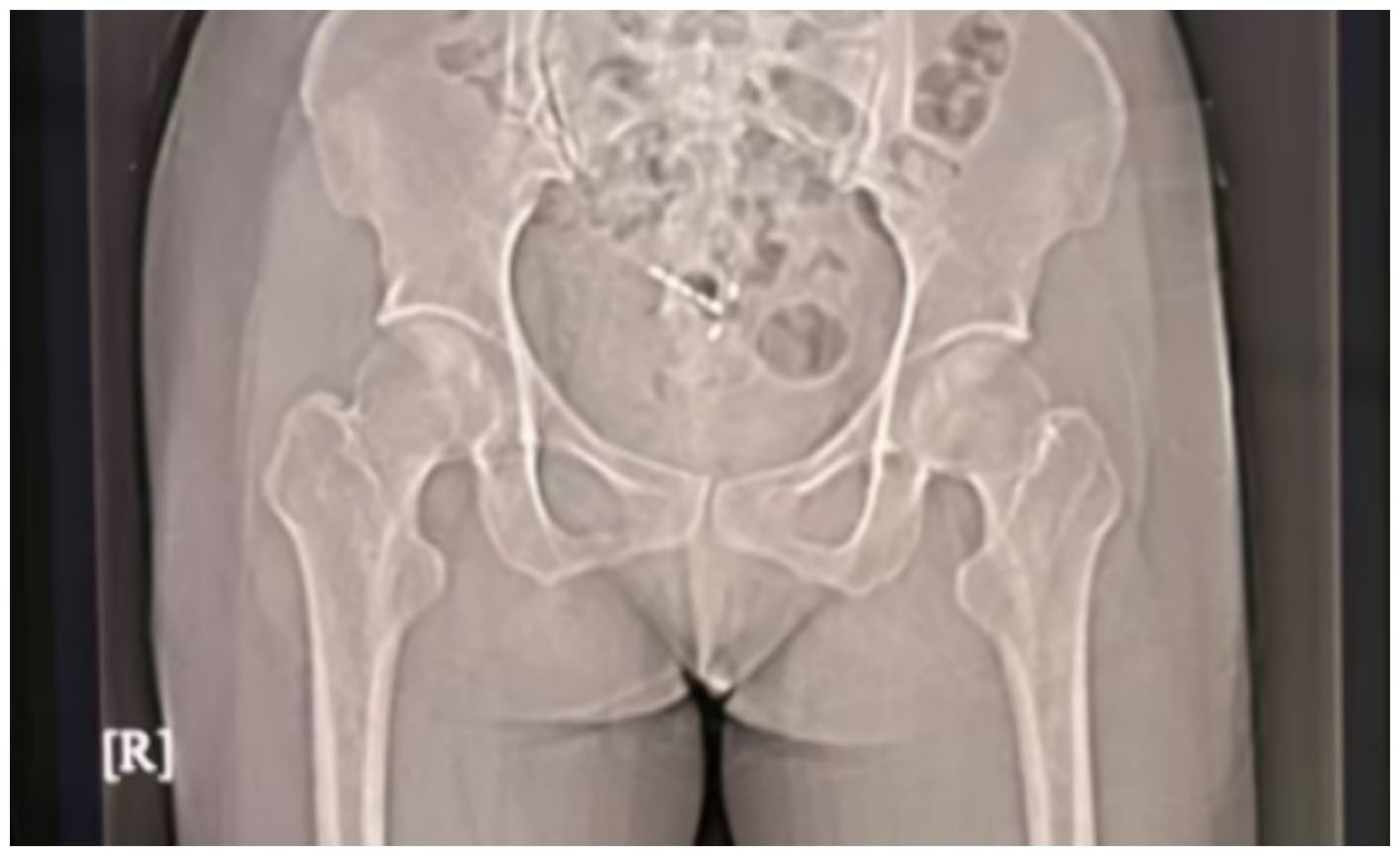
Preoperative pelvic X-ray image.
